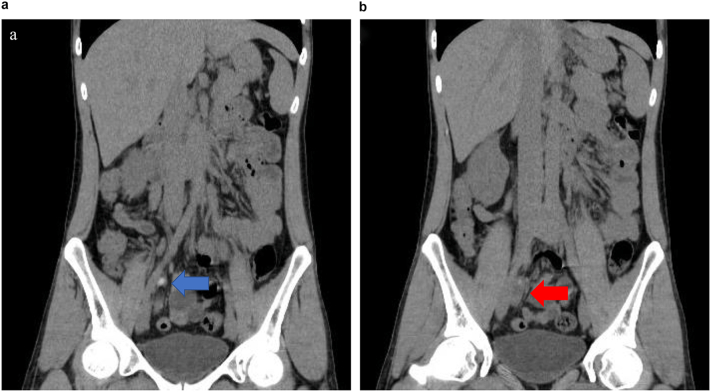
Computed tomography findings. (A) Appendicolith (blue arrow) was found in the appendix. (B) A swollen appendix was noted (red arrow). (For interpretation of the references to color in this figure legend, the reader is referred to the web version of this article.) (For interpretation of the references to color in this figure legend, the reader is referred to the web version of this article.)
